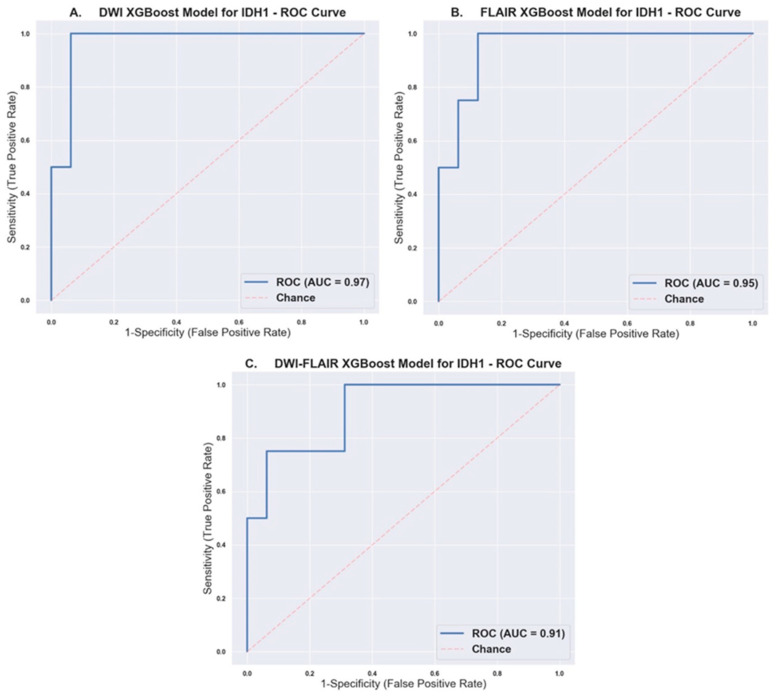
Receiver Operating Characteristic Curves with calculated AUC for (A) Diffusion-Weighted-Imaging (DWI), (B) Fluid-Attenuated Inversion Recovery (FLAIR), and (C) DWI-FLAIR XGBoost models.
